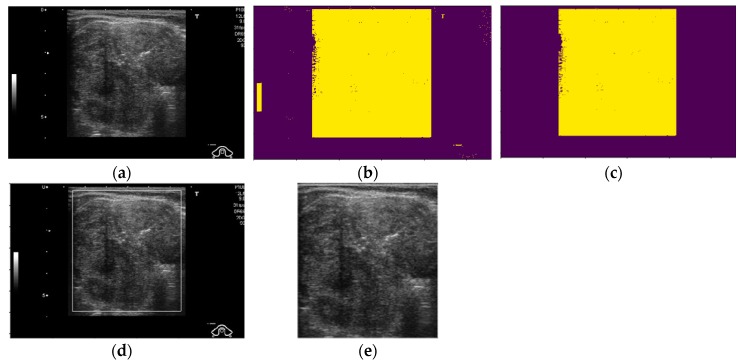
Sample result of our thyroid region ROI extraction method: (a) input captured ultrasound thyroid image with additional background and artifacts, (b) binarized image, (c) detection of largest object using labeling method, (d) final detected (localized) thyroid region, and (e) extracted thyroid region image.
